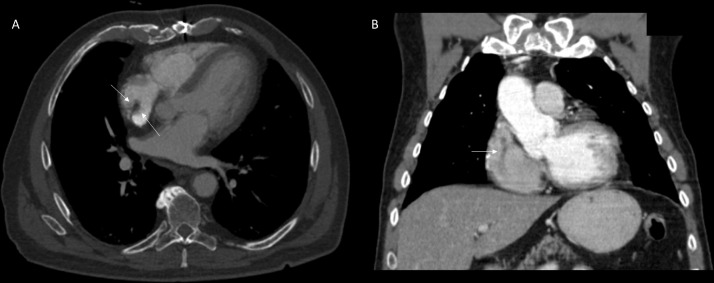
Figure 3: PET/CT examination. MRI cardiac morphology when compared to prior CT component of the PET/CT examination showed classic characteristic findings of crista terminalis. Axial (3A) and Coronal (3B) images show crista terminalis along the lateral wall of the Right atrium. Coronal image shows the complete craniocaudal extent of the crista terminalis (arrow)
